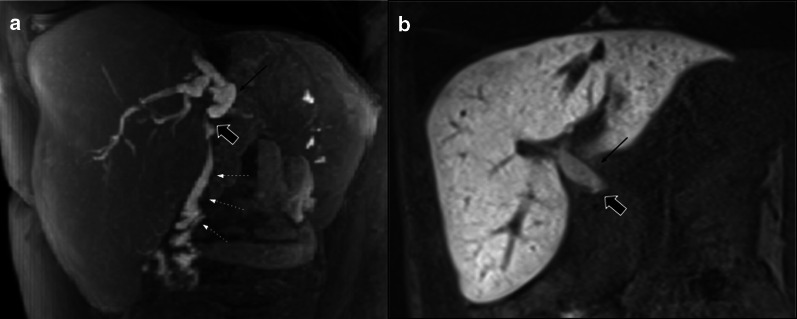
Hepatobiliary-specific contrast agent in hepaticojejunostomy assessment, Hepaticojejunostomy Permeability. Two different patients who underwent hepato-biliary surgeries with hepaticojejunostomy confection. MRI with hepatobiliary-specific contrast agent Gd-EOB-DTPA (gadoxetic acid) was performed due to postoperative biliary complication suspicion. (a, b) Hepaticojejunostomy (thick black arrows) permeability evaluation: MR Cholangiographic image shows afferent limb opacification (dotted arrows in a) that confirmed permeability, while stop in hepaticojejunostomy during hepatobiliary late phase (i.e., 5 h after IV agent injection) depict anastomosis stenosis. Common bile duct (thin black arrow)
